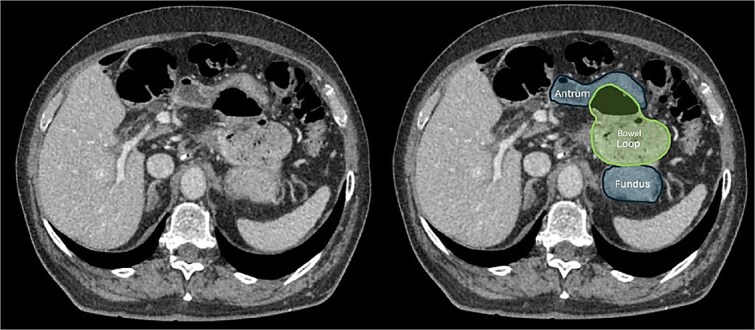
CT scan of the abdomen showing that the colon passing above and to the left of the lesser curvature of the stomach, implying that it had herniated through the foramen of Winslow and into the lesser sac.
